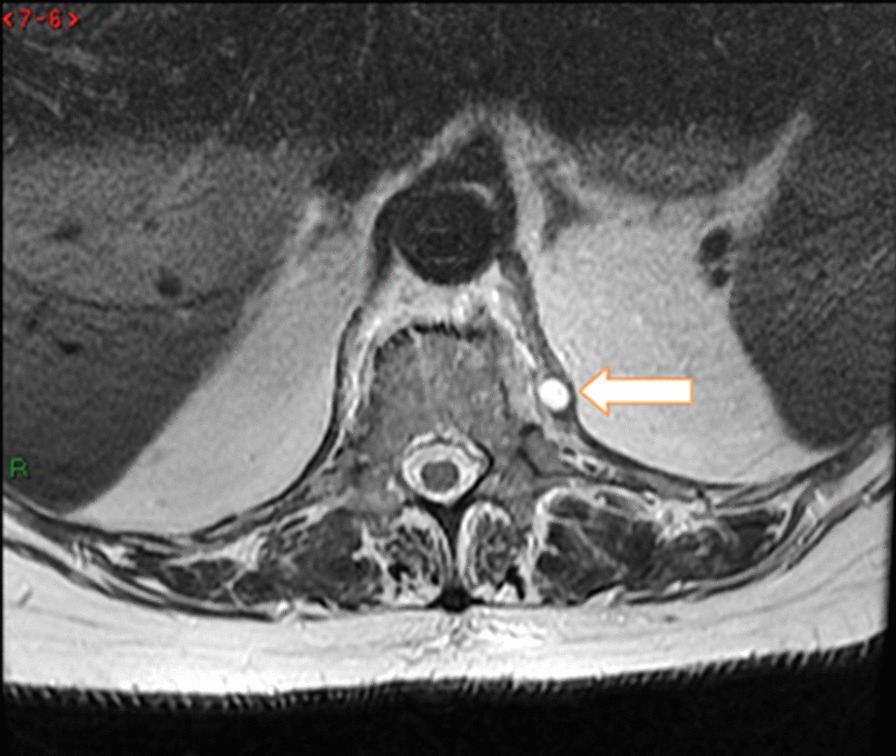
Axial T1 fat-saturation (FS) injected magnetic resonance imaging showing a left paravertebral collection adjacent to T12 (arrow)
